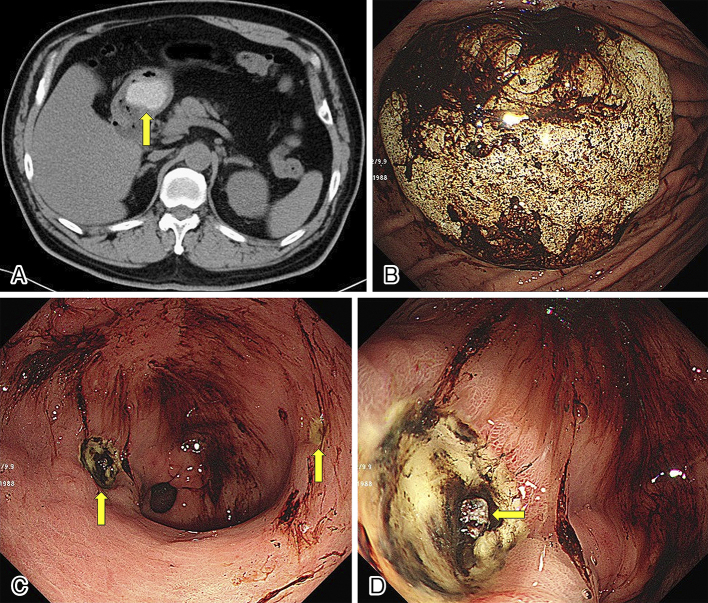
(A) Abdominal computed tomography scan showing a high-density mass (arrow) in the stomach. (B) Esophagogastroduodenoscopy (EGD) showing a whitish solid structure with hematin in the gastric body. (C) EGD showing two gastric ulcers (arrows) and hematin in the antrum. (D) One of the gastric ulcers had a visible vessel (arrow), and hemostatic clips were applied.
